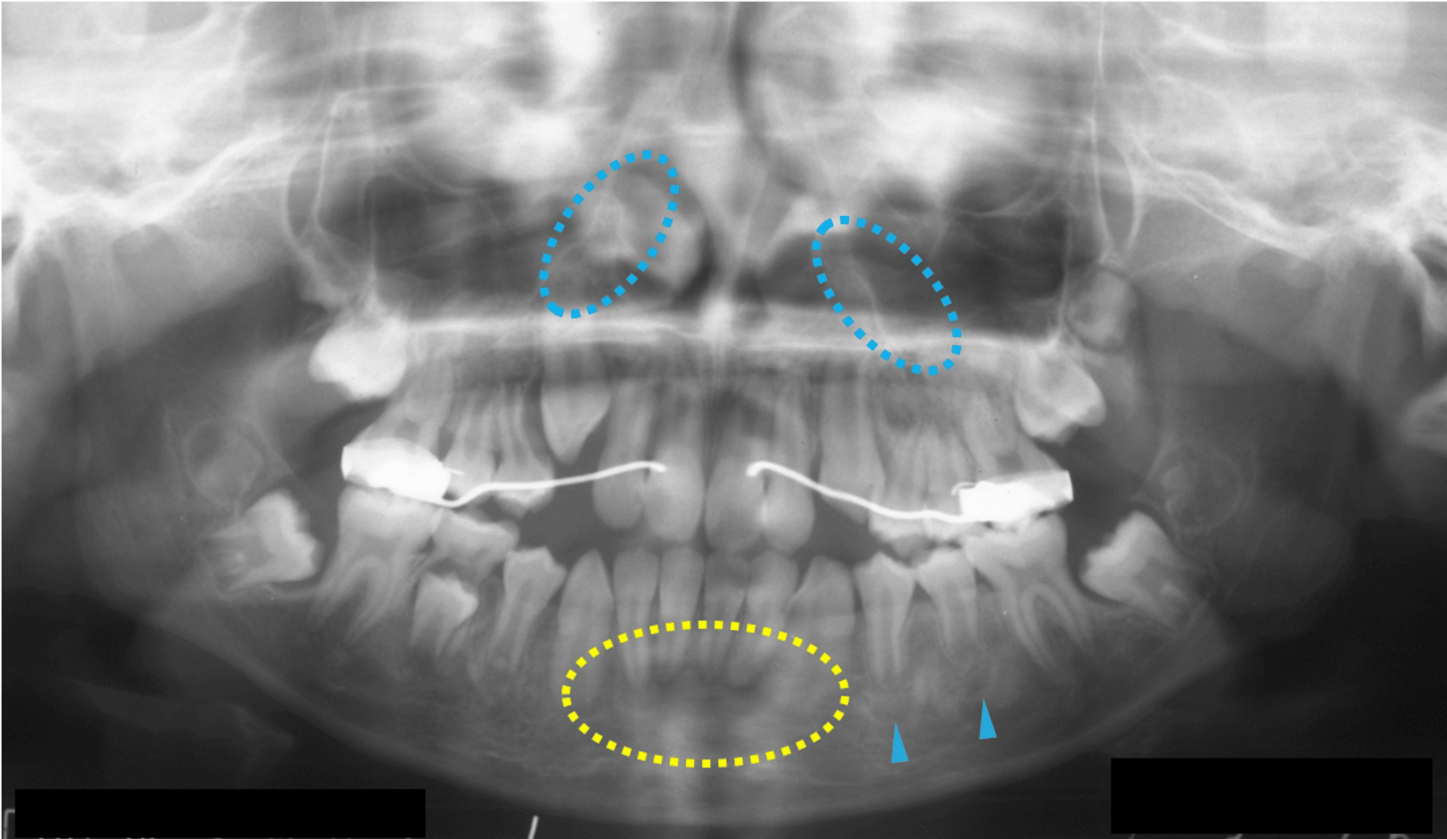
Maxillofacial radiograph at age 10 Blue dotted circles indicate a cotton-like radiopaque image of cemento-osseous lesions in the maxillary sinus. Blue arrowheads indicate opacities in the mandible bone areas encircling the roots of the premolar teeth. Yellow dotted circles indicate a radiolucent lesion in the mandibular bone area apical to the incisors
